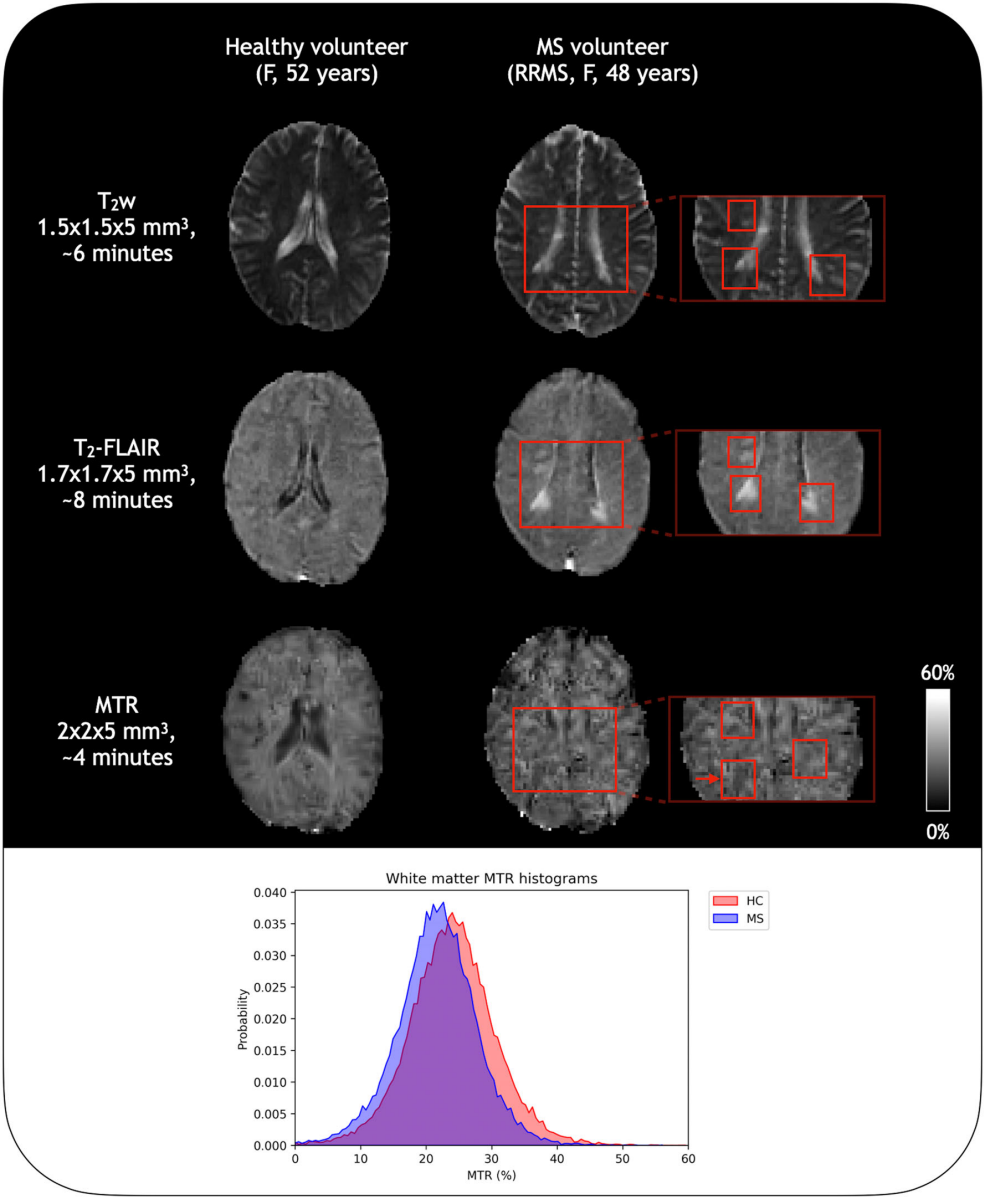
Magnetization transfer ratio (MTR) differences between a multiple sclerosis (MS) and age‐matched healthy volunteer. Red boxes on the images mark out visible lesions in the MS volunteer's T_2_‐weighted and T_2_‐FLAIR images that are also visible on the MTR map despite lower resolution. The MTR white matter histograms show lower MTR values overall in the MS volunteer than the healthy volunteer, which would be expected for diffuse demyelination. The lesion marked by the arrow showed a substantially lower MTR (16.9 ± 3.7%) than the normal‐appearing white matter (21.5 ± 6.2%). The somewhat patchy appearance of the MTR maps may be related to post‐processing steps. The MTR map of the healthy volunteer shows slightly higher values adjacent to the ventricles, likely due to a combination of ventricle pulsation, flow effects, and partial voluming with surrounding tissue. The MTR map of the MS participant shows cerebrospinal fluid values similar to white matter, likely due to partial voluming of the relatively narrow ventricles with the surrounding white matter. [FLAIR, fluid‐attenuated inversion recovery; T_2_w, T_2_‐weighted.]
